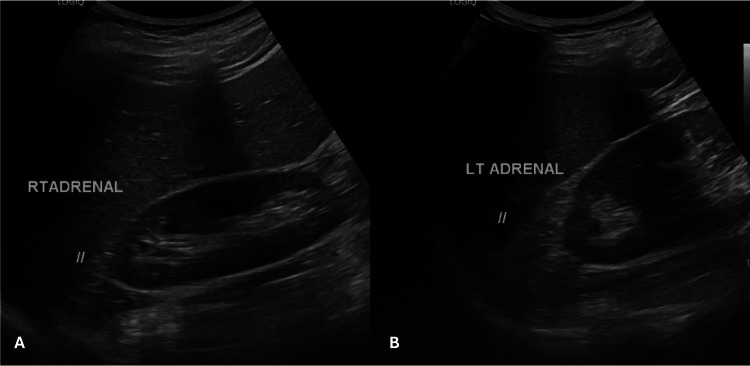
Abdominal ultrasound demonstrating bilateral adrenal enlargement in a patient with CAH, consistent with chronic adrenocorticotropic hormone stimulation. (A) Right adrenal gland showing enlargement (//); (B) Left adrenal gland showing enlargement (//). CAH: congenital adrenal hyperplasia
